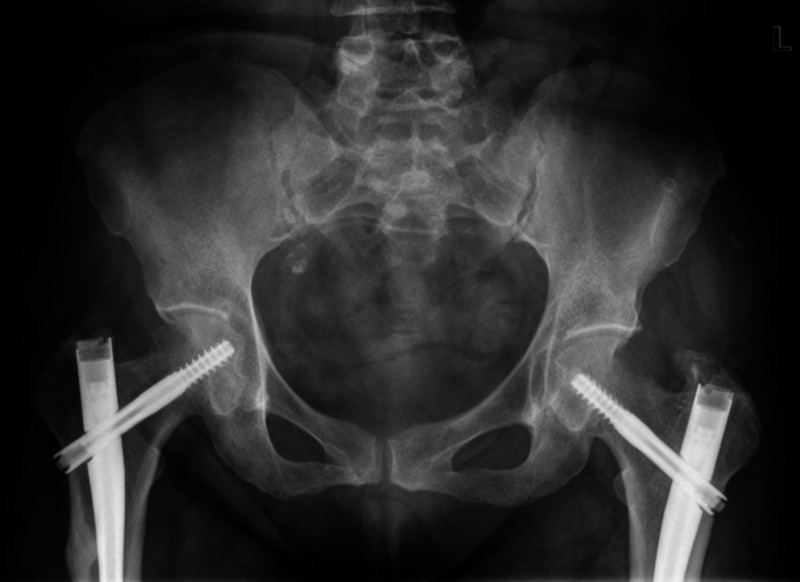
Pelvic X-ray shows intramedullary nail fixation of bilateral atypical femoral fractures
